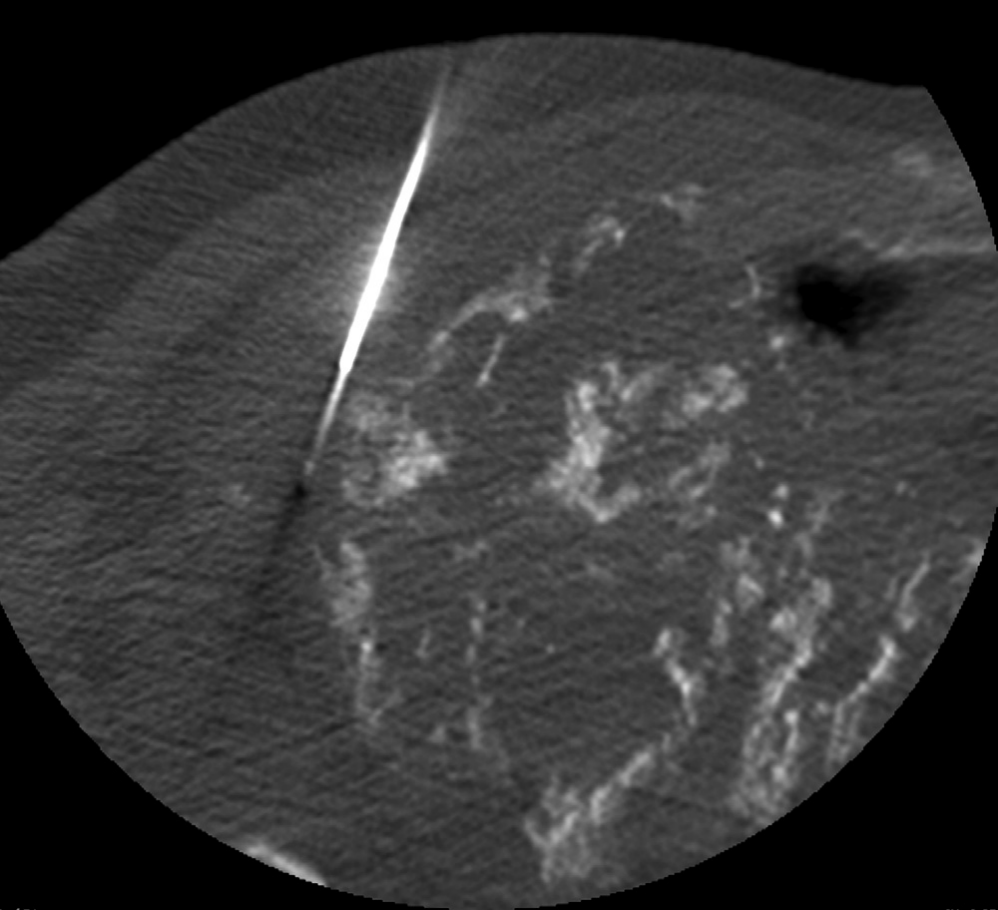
CT-guided 16-gauge core biopsy of right upper lobe lung mass soft tissue component was obtained
